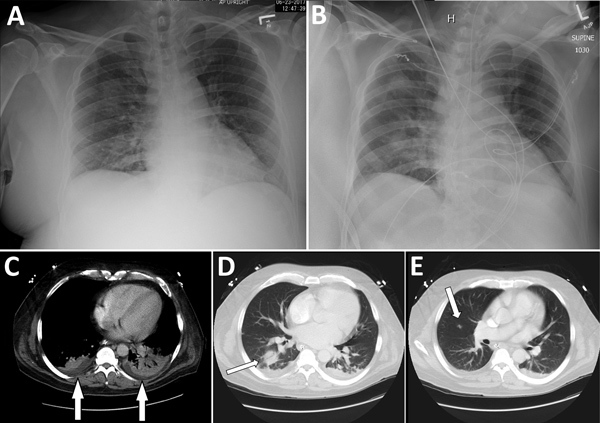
Radiographs (A, B) and computed tomography (C–E) images of chest of organ donor with *Francisella tularensis* infection, United States, 2017. Computed tomography images were taken after brain death. A) Anteroposterior view with patient in upright position, taken on day of admission; B) anteroposterior view with patient in supine position, taken on hospital day 10. C) Small bibasilar pleural effusions with adjacent subsegmental atelectasis versus pneumonia in the lower lobes (arrows); D) 3-cm round focus of pneumonia in the right lower lobe (arrow); E) 1-cm ill-defined nodule in the inferior right upper lobe (arrow).
